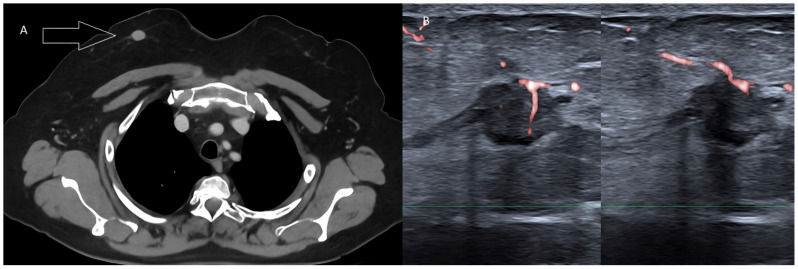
Pure tubular carcinoma detected incidentally during whole-body CT in a female patient with rectal cancer. (A) Contrast-enhanced, venous-phase CT scan detecting a nodule within the right breast (arrow). (B) Targeted US scan displaying the malignant lesion.
